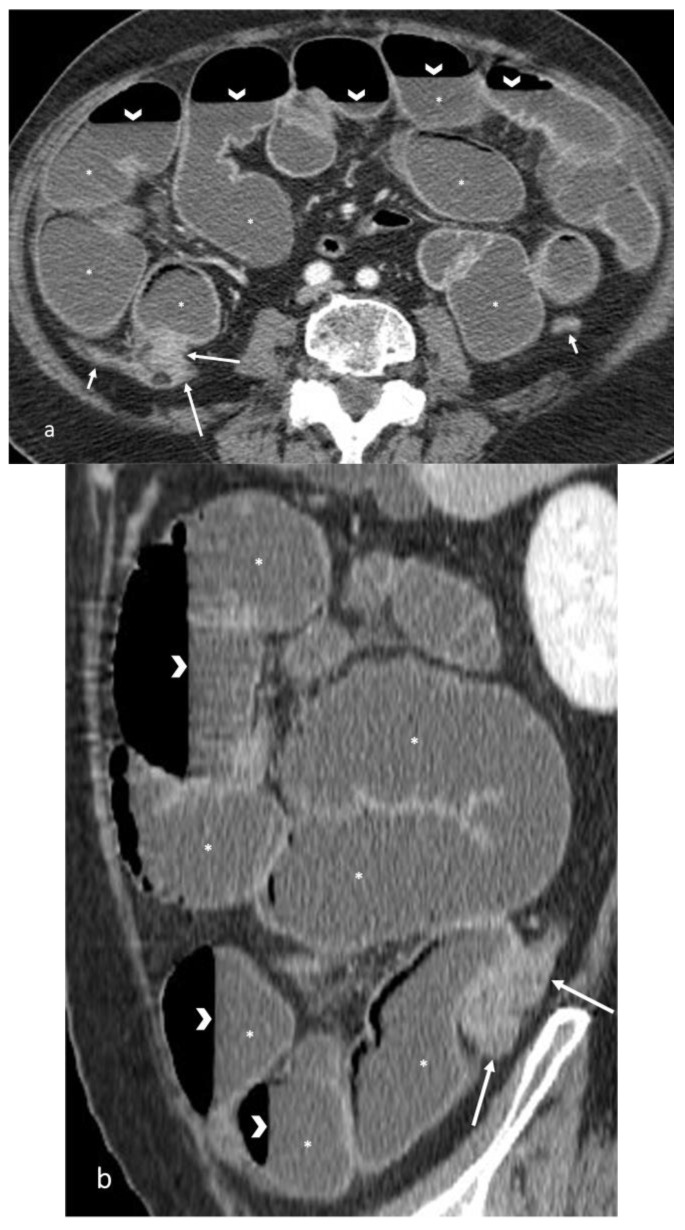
CT appearance of bowel obstruction caused by bowel cancer. (a) Axial and (b) sagittal CT images after contrast medium injection, performed at the emergency department, show irregular bowel wall thickening (long arrows), involving the bowel at the ileocecal valve, related to bowel cancer, causing marked dilatation of upstream bowel loops (asterisks) with multiple air–fluid levels (arrowheads). The downstream colon loops are collapsed (short arrows).
